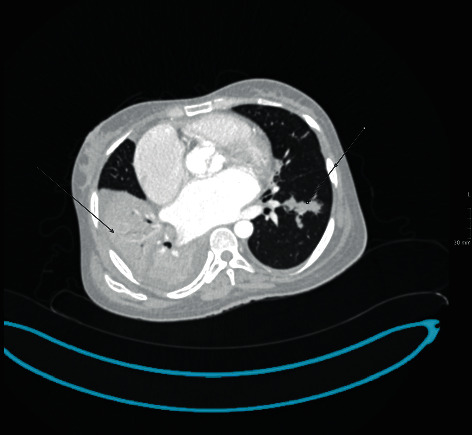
CT chest axial image showing bilateral lung consolidations.
